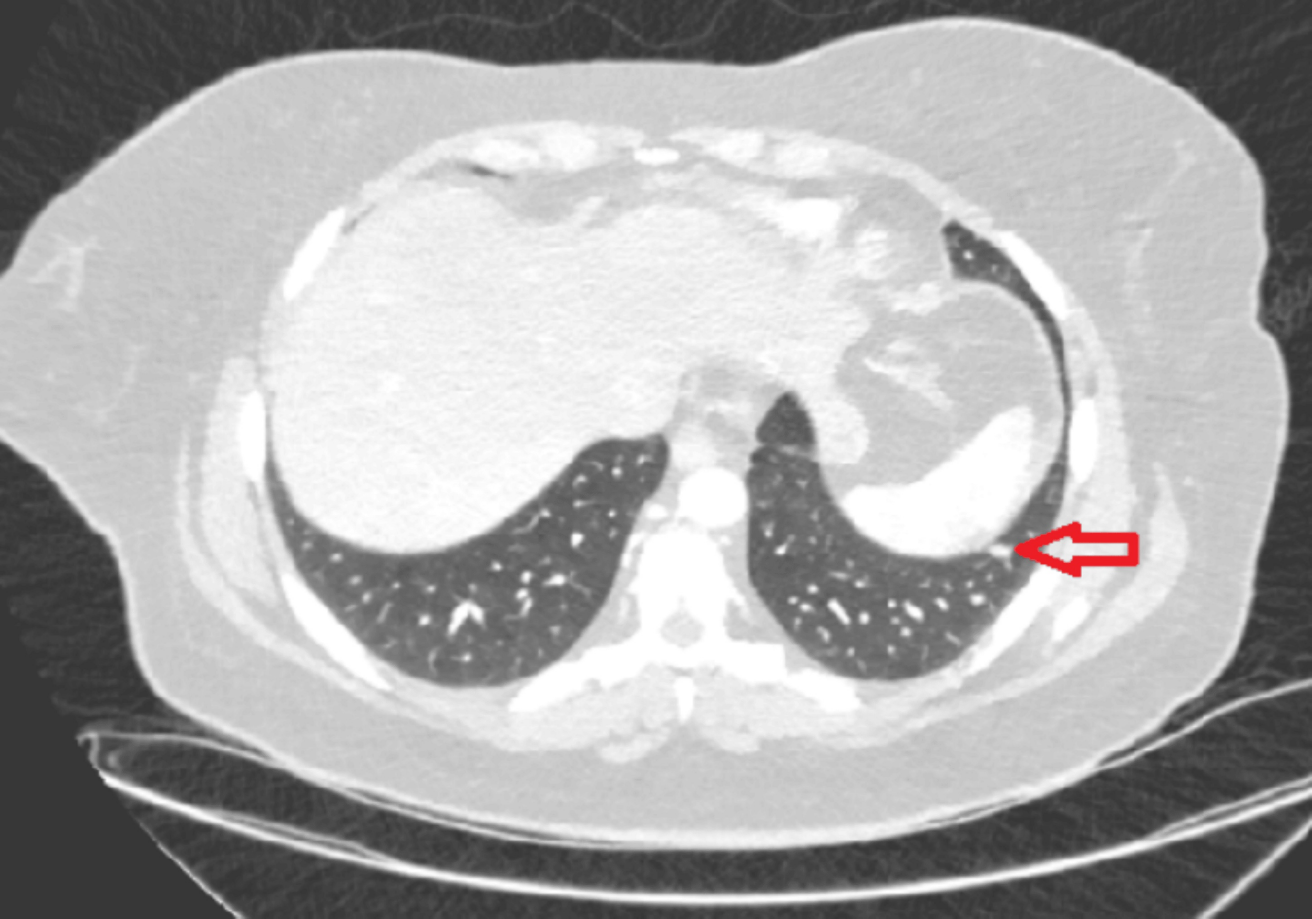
Axial CT scan of the chest demonstrating a small subpleural nodule measuring 5-6 mm in the left lower lobe (red arrow).
